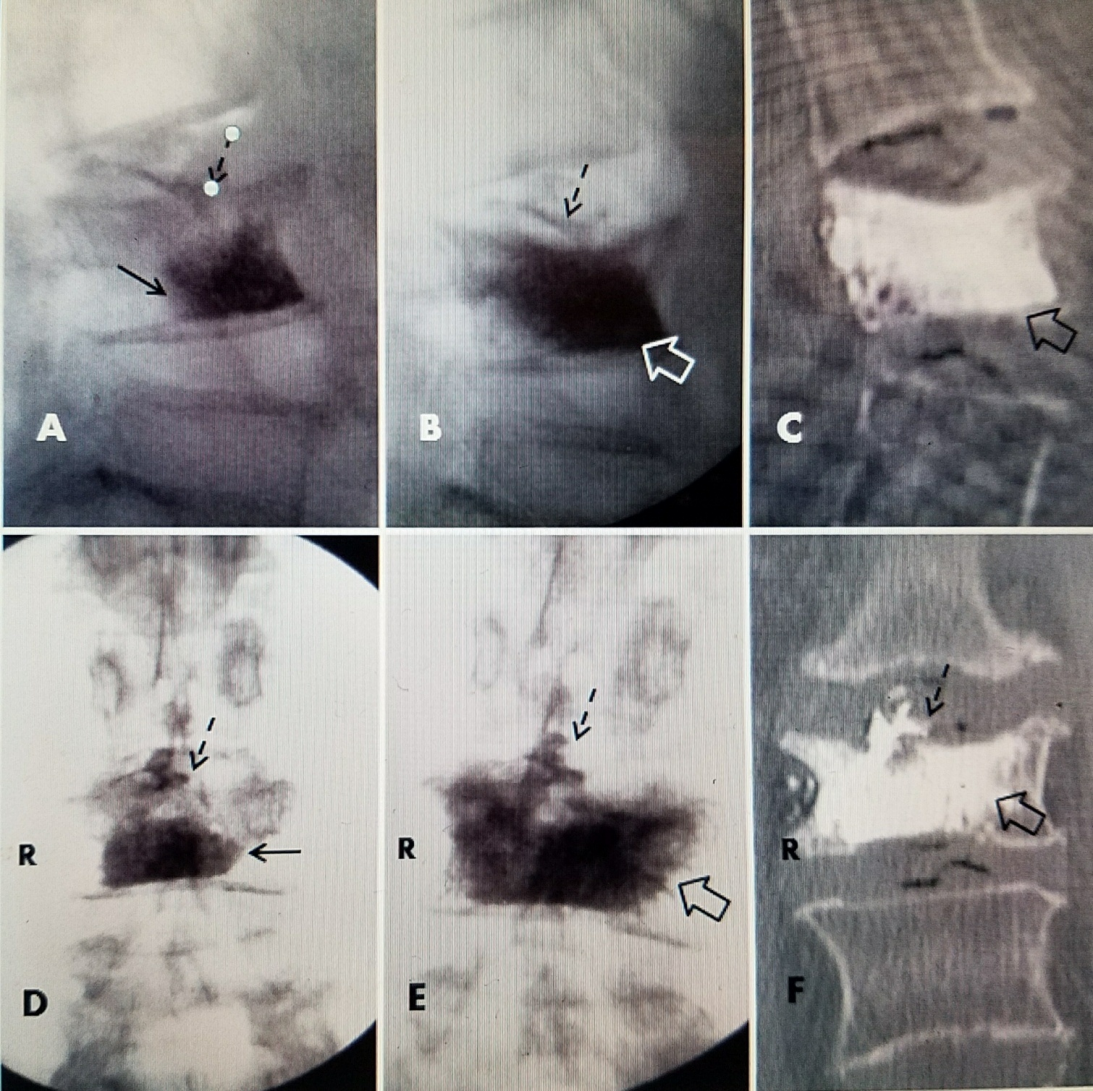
L4 kyphoplasty inferior and left and after bilateral vertebroplasty A: Initial intra-operative films of vertebral augmentation with balloon kyphoplasty using polymethylmethacrylate (PMMA): Lateral fluoroscopic image showing cement is only in the anterior and inferior body of L4 (solid black arrow) but definitely below and not in contact with the fracture of the superior endplate where a small amount of cement has extravasated (dotted black arrow) B: Lateral image after second vertebral augmentation using Cortoss® showing diffuse fill of the entire vertebrae, including the area adjacent to the fracture at the superior endplate (hollow white arrow). The intra-discal extravasation is unchanged from the first procedure (dotted black arrow) C: Sagittal computerized tomography (CT) after repeat vertebroplasty showing complete and diffuse fill of the vertebral body, including under the entire superior endplate and posterior wall of L4 (hollow black arrow). D: Anterior-posterior (AP) film after initial kyphoplasty showing only partial fill in the right inferior part of the vertebrae. There is minimal spread of cement below the fractured superior endplate (solid black arrow). There is intradiscal extravasation into the disc space at L3-4 (dotted black arrow). E: AP image after second procedure showing diffuse bilateral Cortoss® cement filling both sides of the vertebrae. There is better fill superiorly across the area of superior endplate fracture and inferiorly on the left (open black arrow). There is no change in the intradiscal extravasation at L3-4 from the first procedure (dotted black arrow). F: AP image of CT scan showing diffuse cement fill of L4 vertebrae (hollow black arrow). The intradiscal extravasation of cement at L3-4 is unchanged (dotted black arrow) from initial extravasation after the kyphoplasty as can be seen comparing images D, E, and F.
